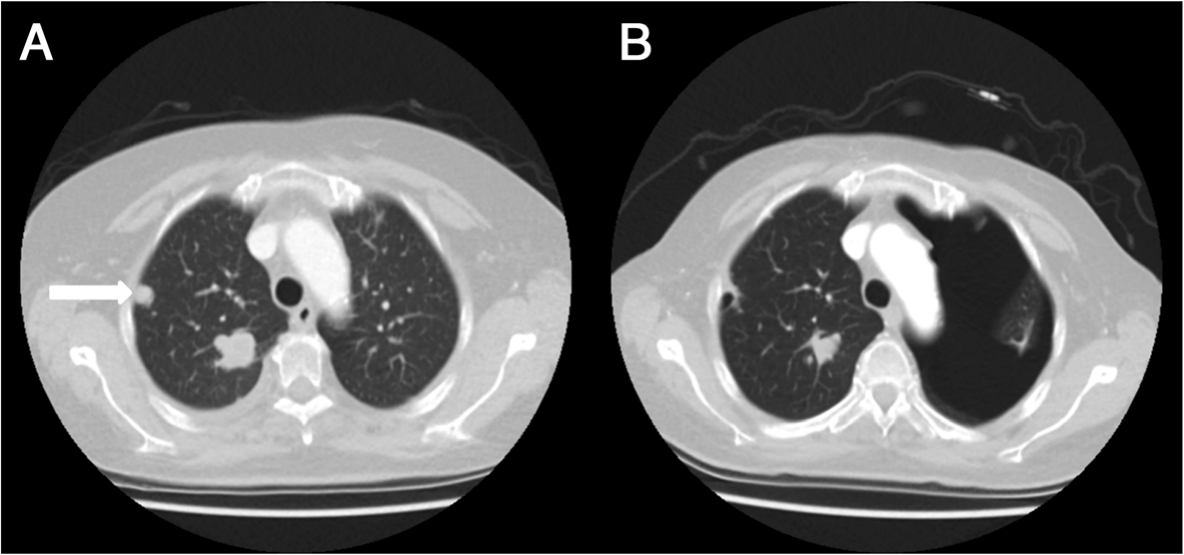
CT scans of patient 5. A: CT scan before the start of pazopanib showing multiple metastases in the right lung, one is indicated by the arrow. B: Routine follow-up CT scan during pazopanib treatment showing the left-sided pneumothorax and the earlier mentioned metastasis in the right lung is now showing cavitation.
